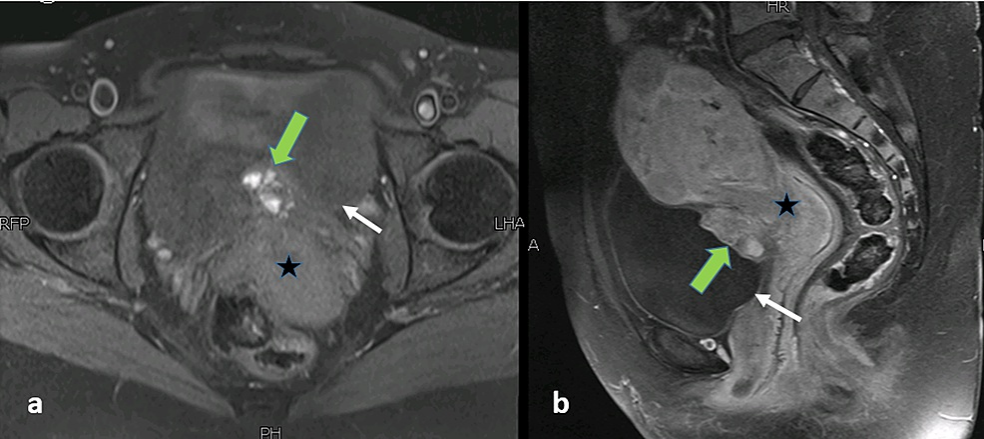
MRI pelvis - image 2 (a) Axial T1-weighted fat-saturated image showing ill-defined heterogeneously hyperintense lesion (green arrow) along the base of the urinary bladder (white arrow). (b) Sagittal post-contrast T1-weighted fat-saturated image showing patchy enhancement within the lesion (green arrow). It is closely abutting the lower uterus (star sign) MRI: magnetic resonance imaging
